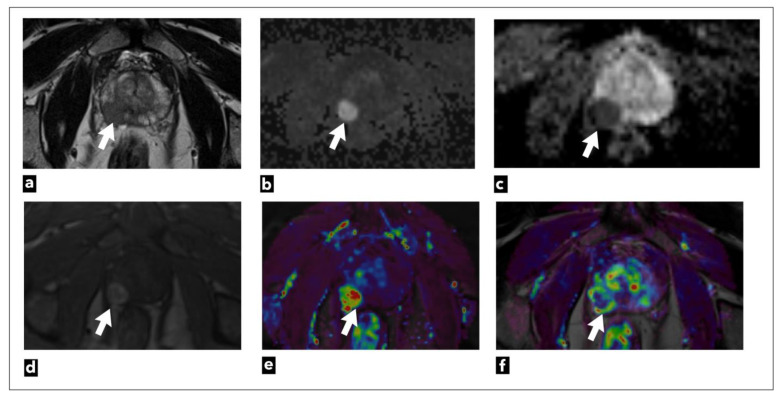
The mpMRI performed on a 75-year-old male patient shows a right peripheral zone prostate lesion (white arrow) that was confirmed to be cancer on biopsy; Gleason score 4 + 3 = 7. The images present the following: (a) T2W as a hypointense focal lesion; (b) DWI b = 1400 s/mm^3^; (c) ADC map demonstrates highly restricted diffusion and early contrast enhancement; (d) raw data; (e) semi-quantitative colour-coded parametric map for a wash-in; (f) pharmacokinetic quantitative colour-coded parametric map for Ktrans.
